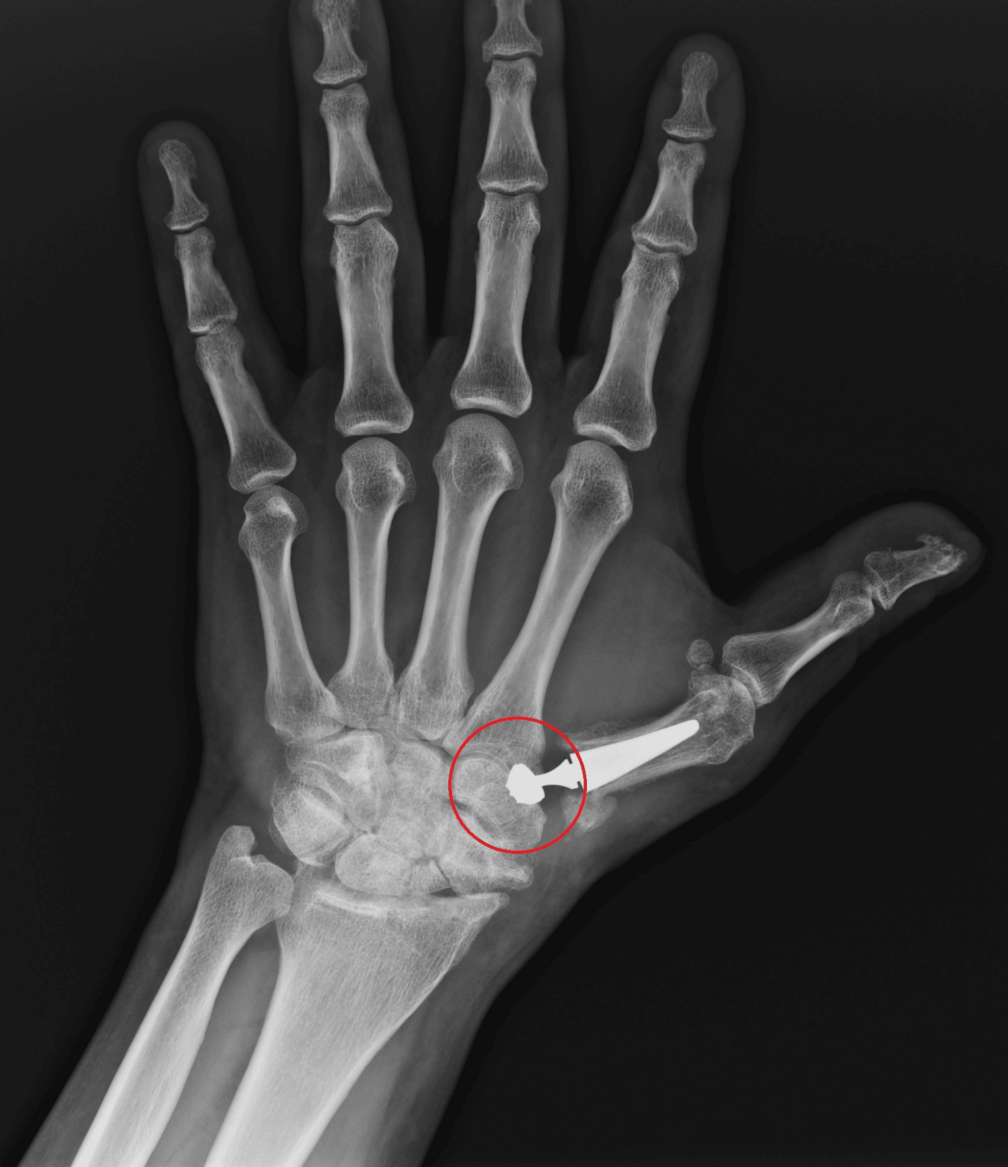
X-ray in the A-P projection obtained two years after surgery The correct positioning of all endoprosthesis elements (head of a double mobile CMC thumb prosthesis and metal acetabular shell) is marked in red. A-P: anterior-to-posterior
